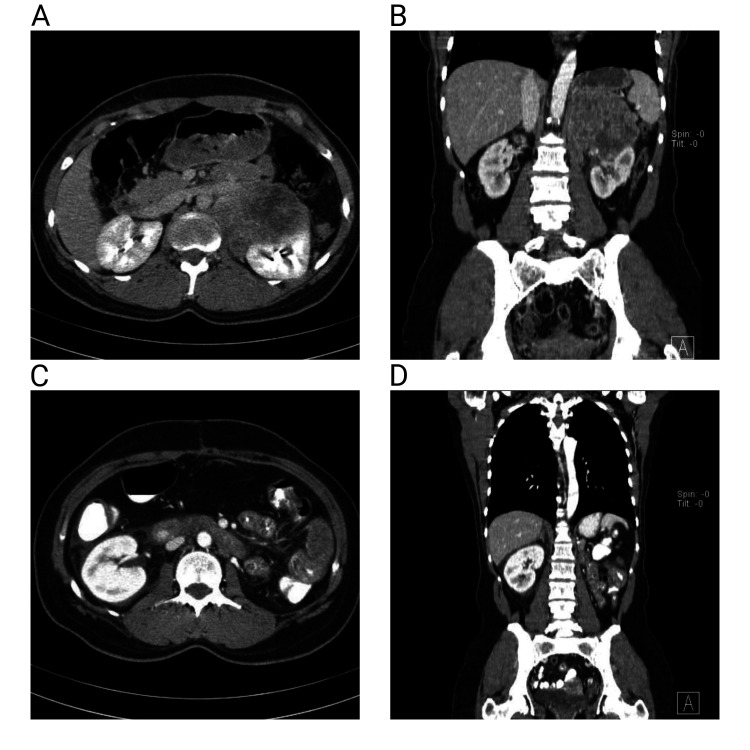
Treatment response assessment by computed tomography (CT) in a patient with left-sided clear cell renal cell carcinoma (ccRC). Pre-treatment scans: (A) axial view showing a large, heterogeneous, well-defined mass originating from the left kidney; (B) coronal view confirming the extent of the left renal mass. Post-treatment scans: (C) axial view and (D) coronal view obtained at similar anatomical levels demonstrate marked interval reduction in the size of the mass, consistent with an effective therapeutic intervention.
